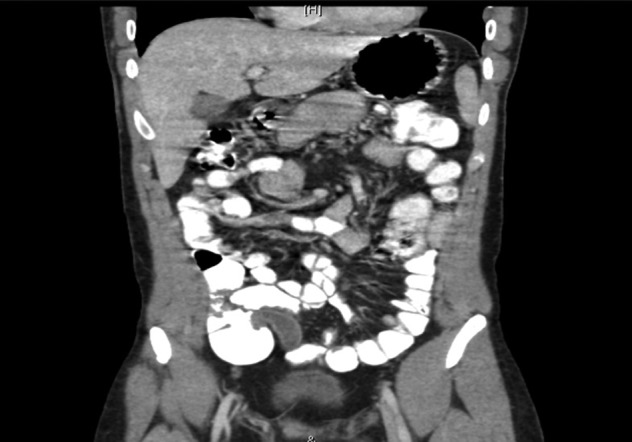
A computed tomography scan of the abdomen showing a dilated appendix with no surrounding fat stranding or free fluid. The wall of the appendix was not interrupted. The liver, spleen, adrenal glands, kidneys, pancreas appeared unremarkable. No free air or free fluid was noted in the abdomen or pelvis. Overall, the findings were consistent with sub-acute appendicitis.
